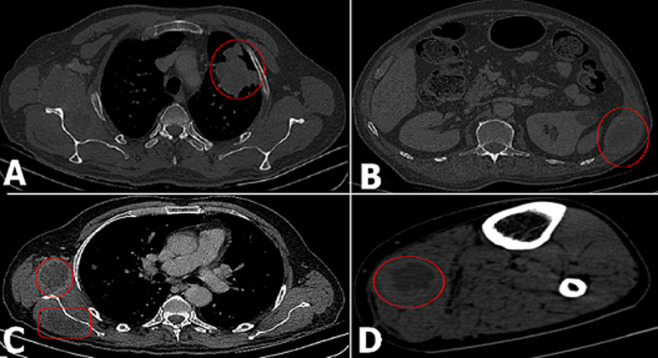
A) thoracic computed tomography (CT) showing a left upper lung mass; B) abdomino-pelvic CT showing a sympathetically maintained pain (SMP) in the left lumbar region; C) SMP shown in the right axillary region; D) CT of the right leg showing a STM in the right calf muscle
